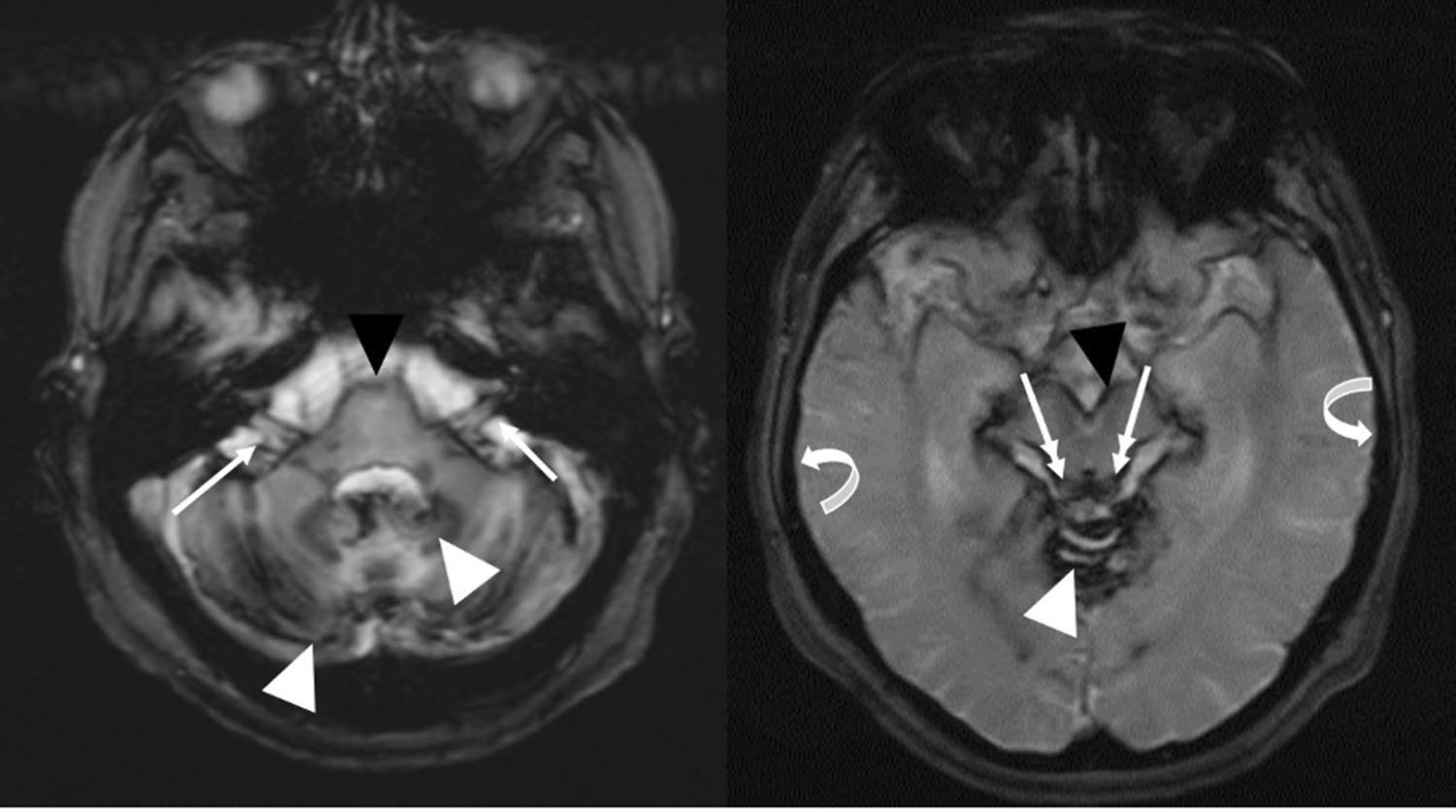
Axial T2*-weighted MRI of Pat XX with superficial siderosis reveals diffuse hypointensity outlining the cortex and nerval tissue in the posterior fossa. A Siderosis of the cranial nerves VII, VIII, IX from their pontine emergence up to the entry in the inner auditory canal (white arrows) and siderosis the cerebellar cortex (white arrow heads). B Siderosis of the quadrigeminal plate (double white arrows) and the upper cerebral vermis (white arrow heads) with sparing of the cortex of both temporal lobes (curved white)
